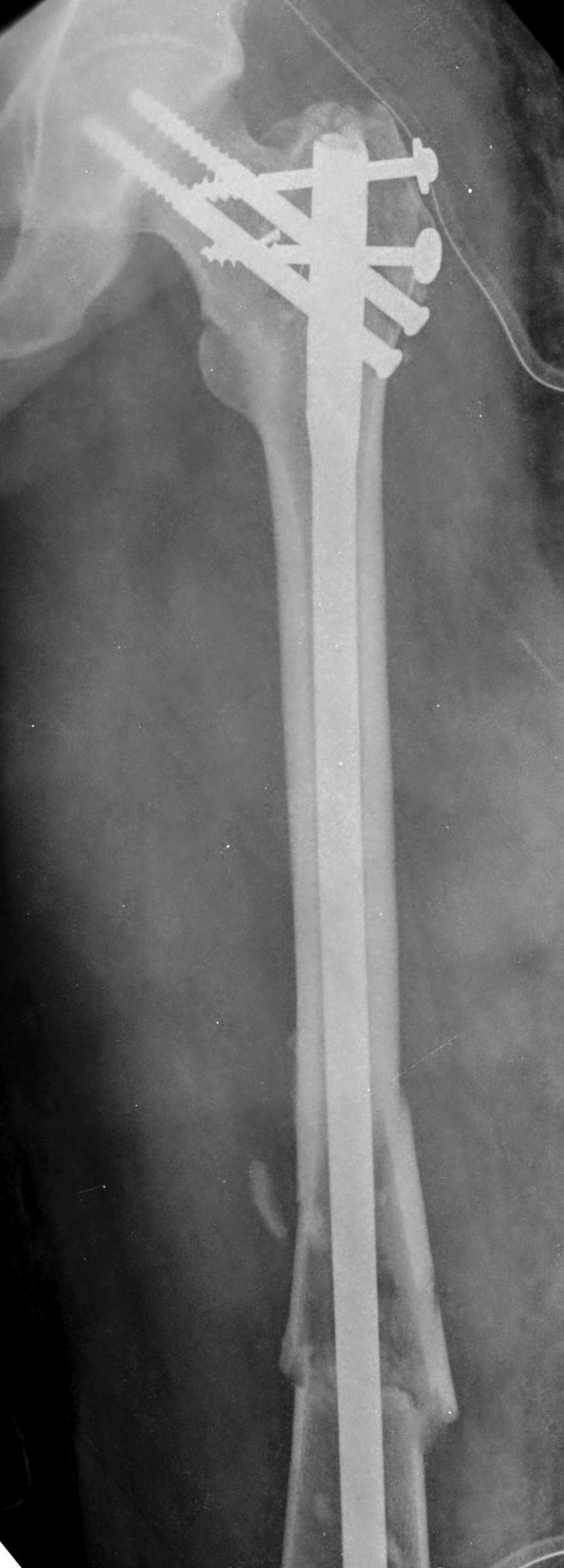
Six months post-op x-ray of the left femur showing union of the left trochanteric and femoral shaft fracture
